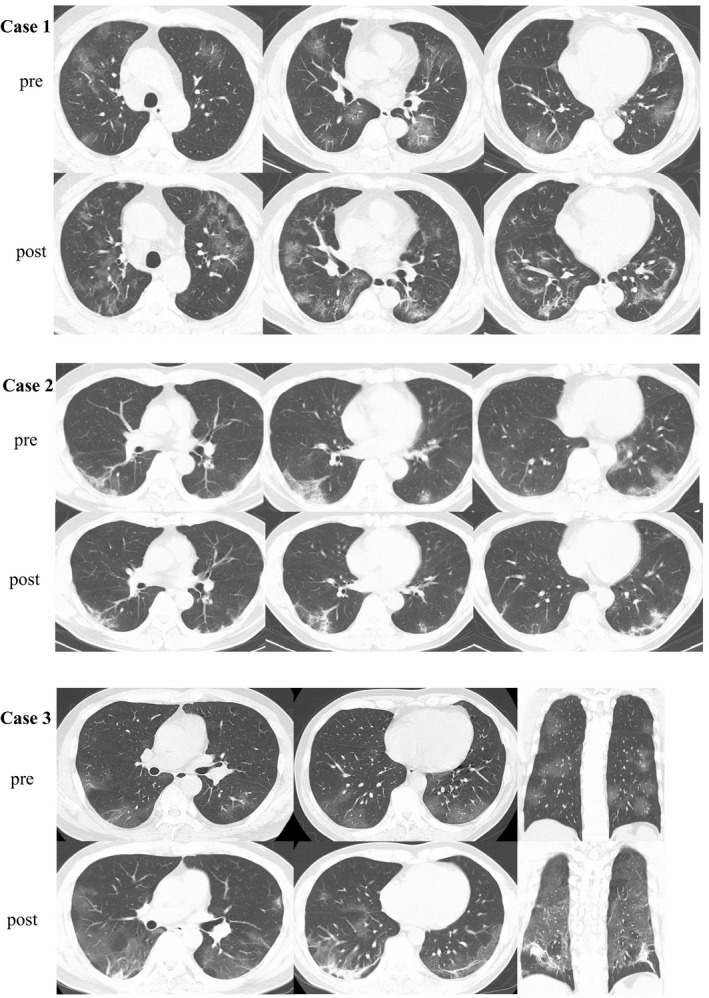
Comparison of CT findings between pre‐ and post‐treatment for COVID‐19 pneumonia. In case 1, the post‐treatment chest CT revealed that GGO in bilateral lungs were increased, furthermore intra‐ and interlobular septal thickening region, bronchiectasis within GGO were emerged. In case 2, the chest CT images reveal bilateral small ground‐glass opacities with linear shadows located dorsally in the lower lung lobes. Post‐treatment chest CT revealed while a part of GGO regions were improved improvement, the linear shadow in GGO was thickened. In case 3, bilateral GGO and septal thickening region were progressed. The regions were predominantly in dorsal. Abbreviation: CT, computed tomography; COVID‐19, coronavirus disease 2019; GGO, ground‐glass opacities
